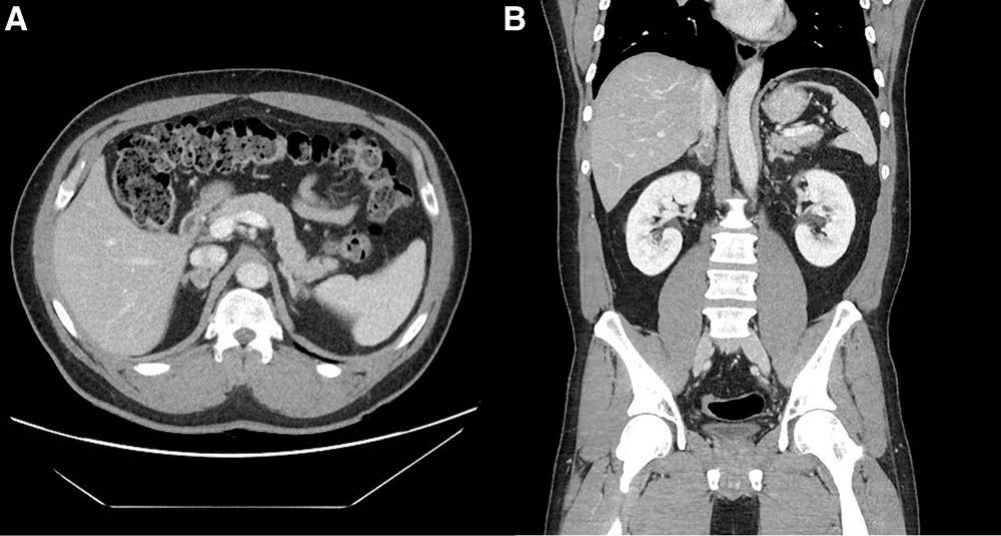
Abdominal CT reveals a 3.5 cm-sized, well-circumscribed, lobulated mass in the right adrenal gland, (A) white arrow on axial image and (B) black arrow on coronal image). On enhanced CT scan, it shows heterogeneous enhancement with punctate calcifications. CT = computed tomography.
